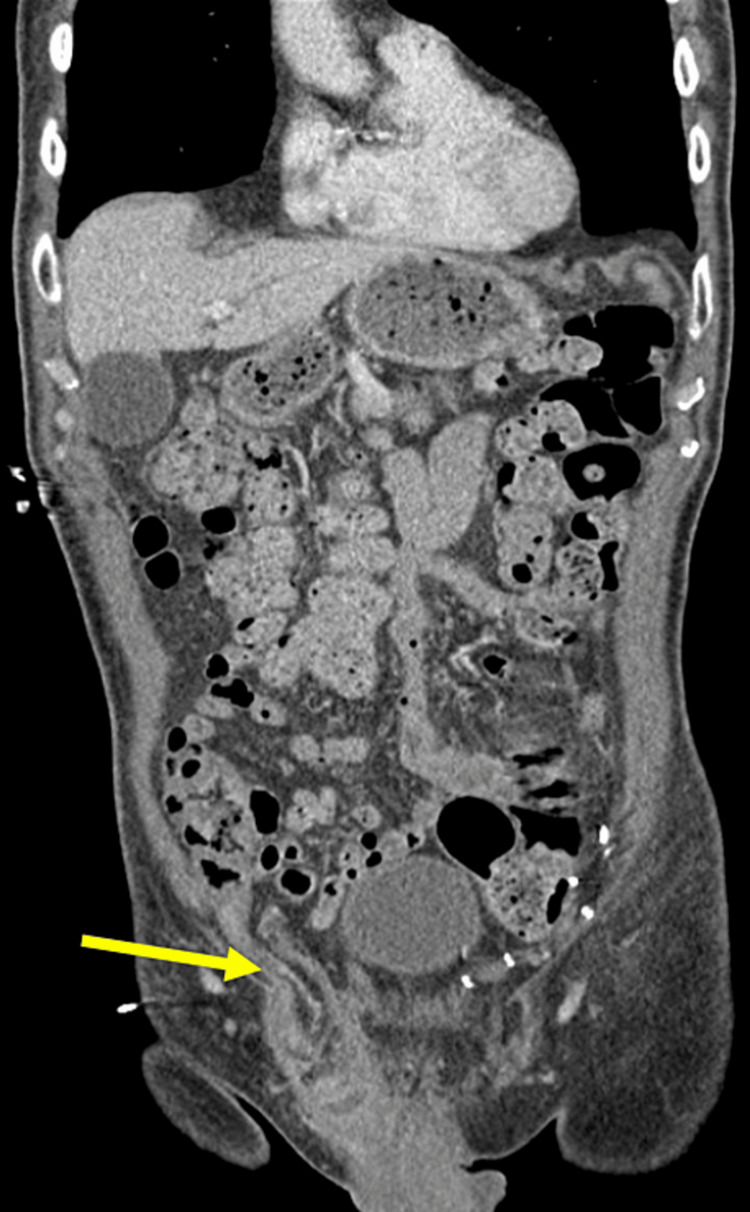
CT abdomen pelvis with IV contrast. Thickened appendix extends into the right inguinal hernia. Interval improvement in the size of fluid collection in the right inguinal hernia with residual collection after placement of a percutaneous pigtail catheter.
